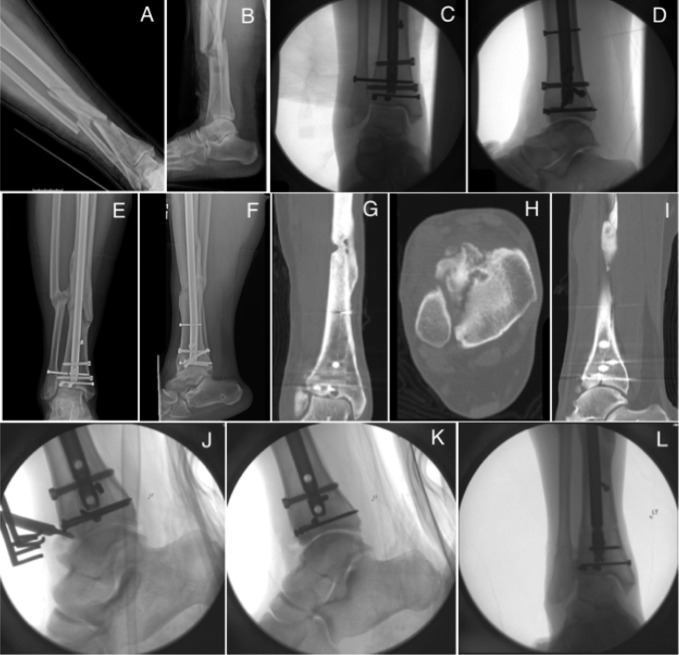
Radiograph demonstrating the (A) AP and (B) lateral injuries of initial injury and subsequent (C) AP and (D) lateral intraoperative fluoroscopic images of initial fixation of an intra-articular distal tibial fracture. Radiographs (E) AP and (F) lateral and CT (G) axial, (H) coronal, and (I) sagittal images after IMN and screw fixation that resulted in a single case of ankle arthrosis. The patient was treated with screw removal and débridement with removal of the anterior osteophyte (J–L).
